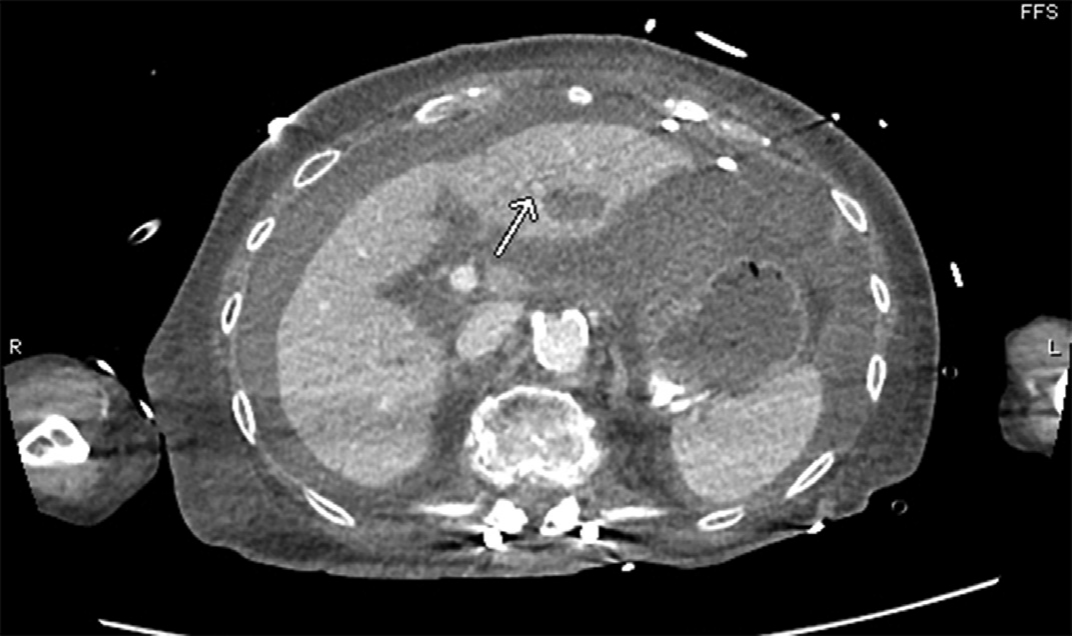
Computed Tomography Angiogram of Torso With Intravenous Contrast Material Enlarged hypoattenuating lesion within left lobe of liver, previously thought to be indeterminate mass, and now favored to represent liver laceration. Arrow points to ill-defined area of hyperattenuation on the arterial phase of the study without definite pooling on delayed phase representing intrahepatic extravasation and pseudoaneurysm formation.
